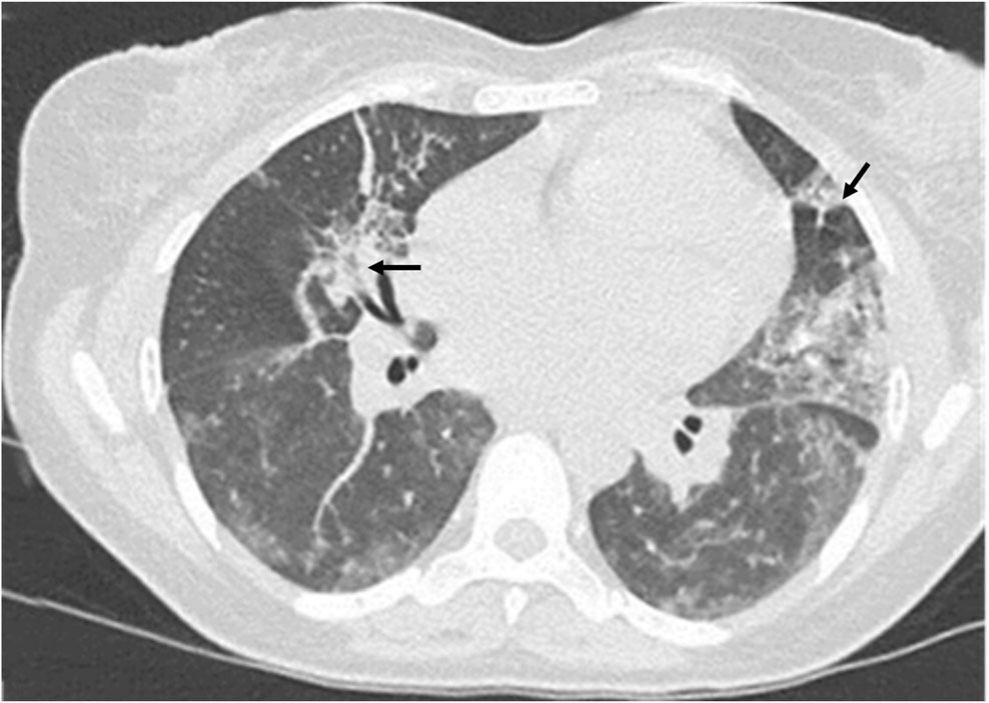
Axial HRCT scan of a patient with H1N1 pneumonia shows bilateral patchy ground-glass opacities associated with small areas of consolidation (black arrows)
